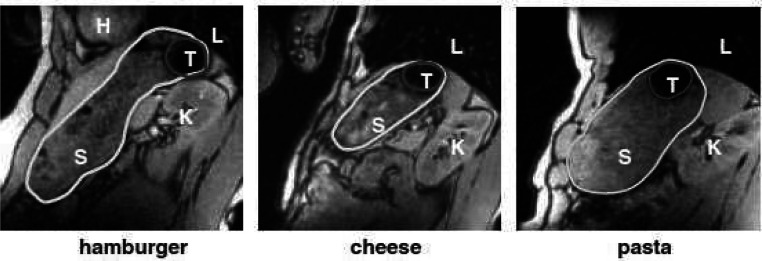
Sagittal MRI images of the stomach taken
